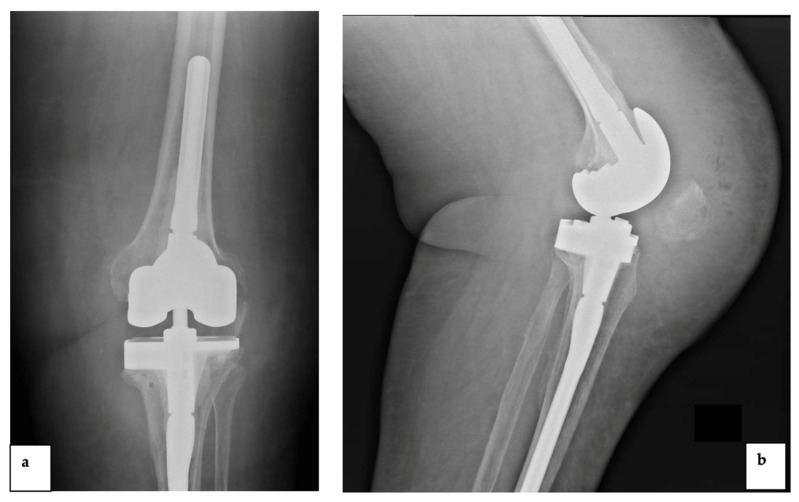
The plain radiograph showed periprosthetic infection of the condylar constrained knee implant due to *Staphylococcus warneri* ((a): anteroposterior view and (b): lateral view).
